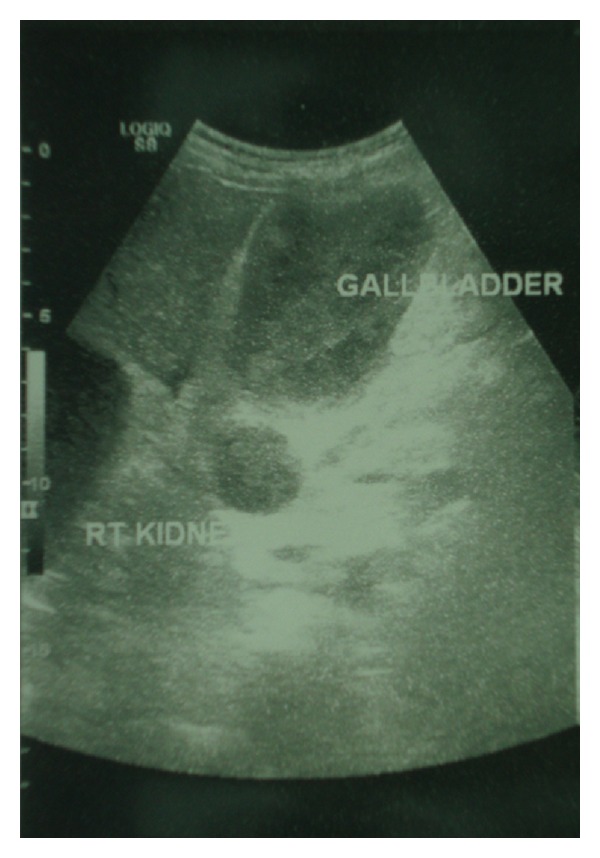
Ultrasound scan demonstrating a distended gallbladder containing echogenic bile (thick sludge or pus or blood-like) without stones and a simple right kidney cyst.
